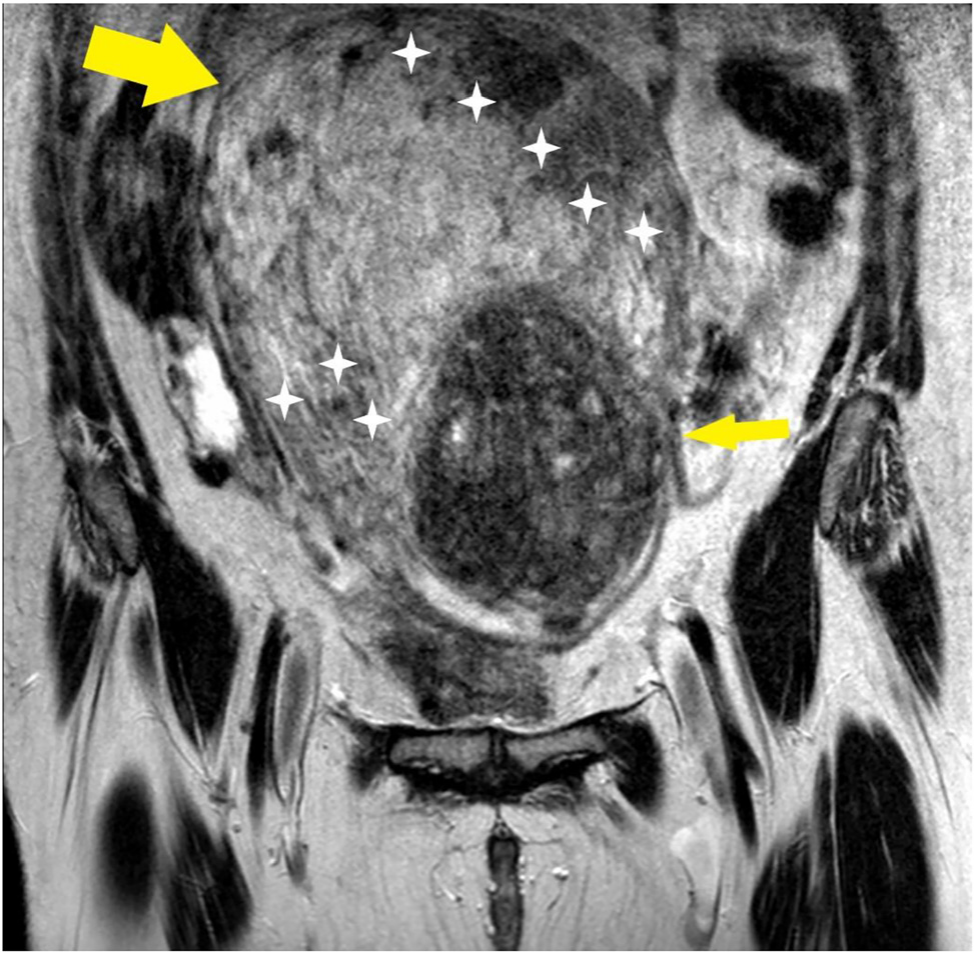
Contrast-enhanced MRI. Coronal T2 weighed acquisition: the uterus is enlarged and shows a hypointense fibroma on the left side (thin arrow). The placenta (delimited by white calipers) is implanted on the right side and marked thinning of the myometrium is evident (large arrow).
